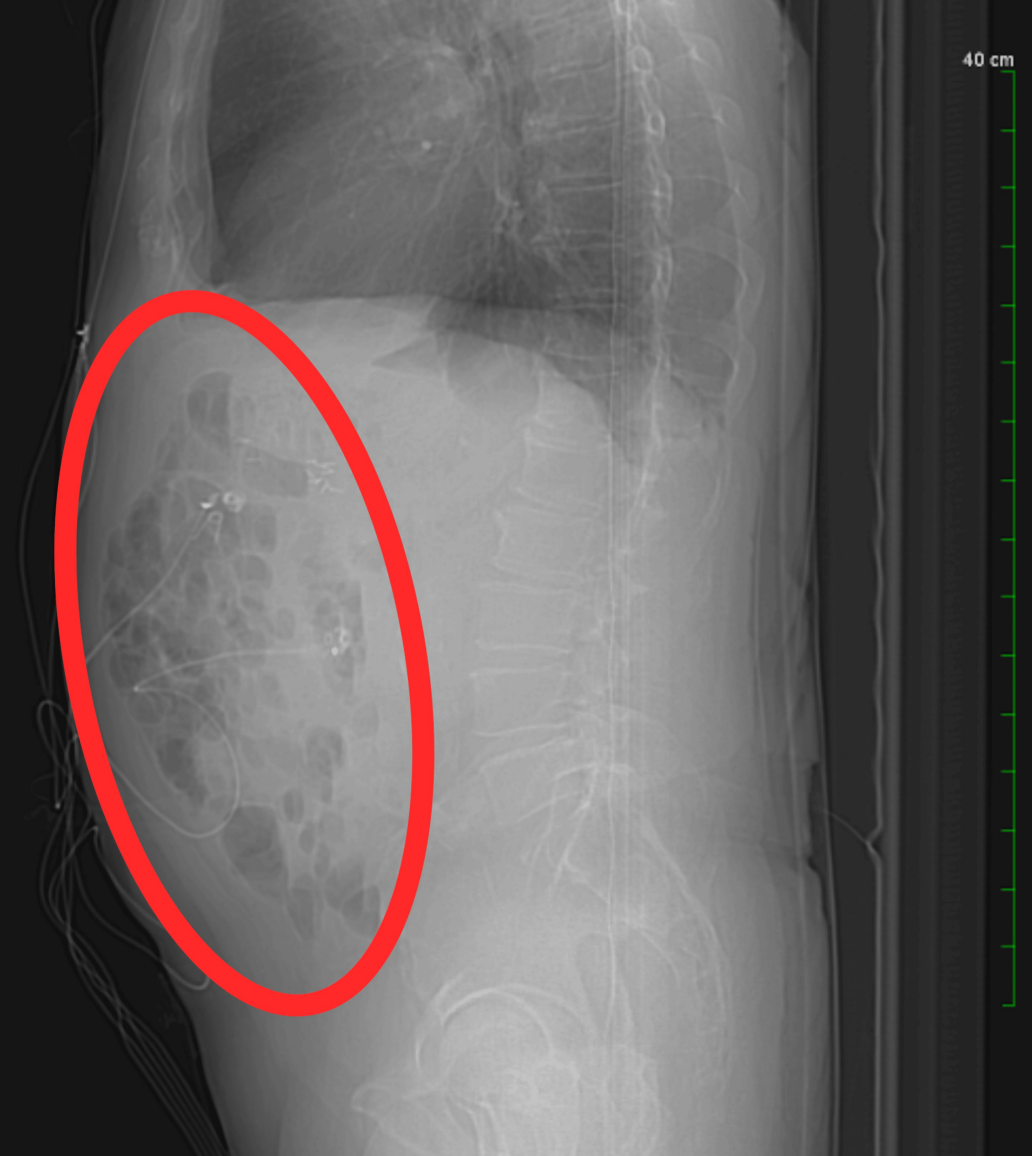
Contrast-enhanced CT scan of the abdomen and pelvis demonstrating multiple air-fluid levels within the colon, consistent with a diarrheal state (red oval). CT: computed tomography
